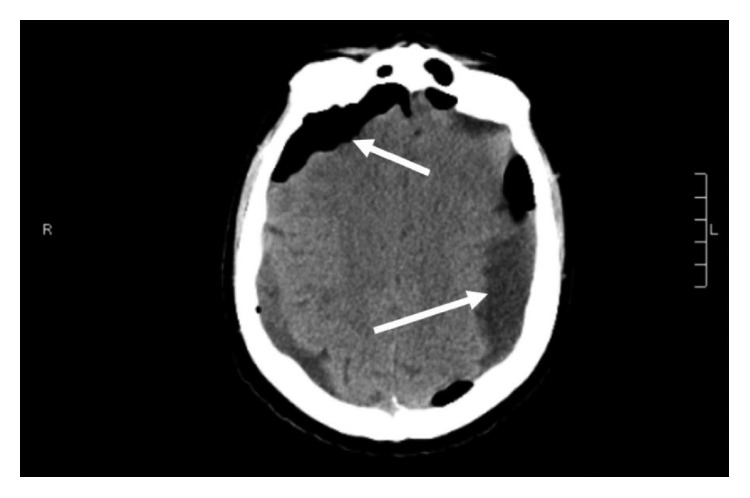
Computed tomography (CT) imaging of the brain after the bilateral burr hole procedure.
